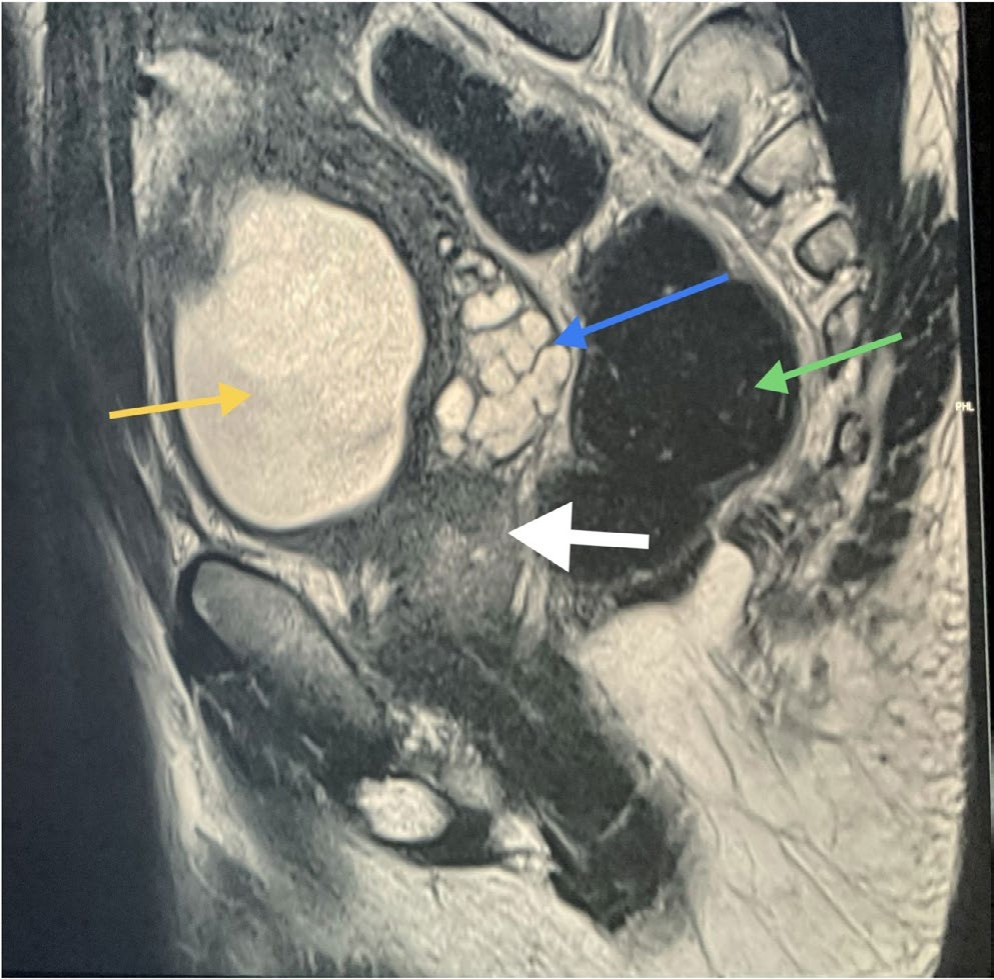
Postoperative MRI Sagittal T2. White arrow (site of resected mass), Yellow arrow (Urinary Bladder) Green arrow (Rectum). Blue arrow (Seminal vesicle)
